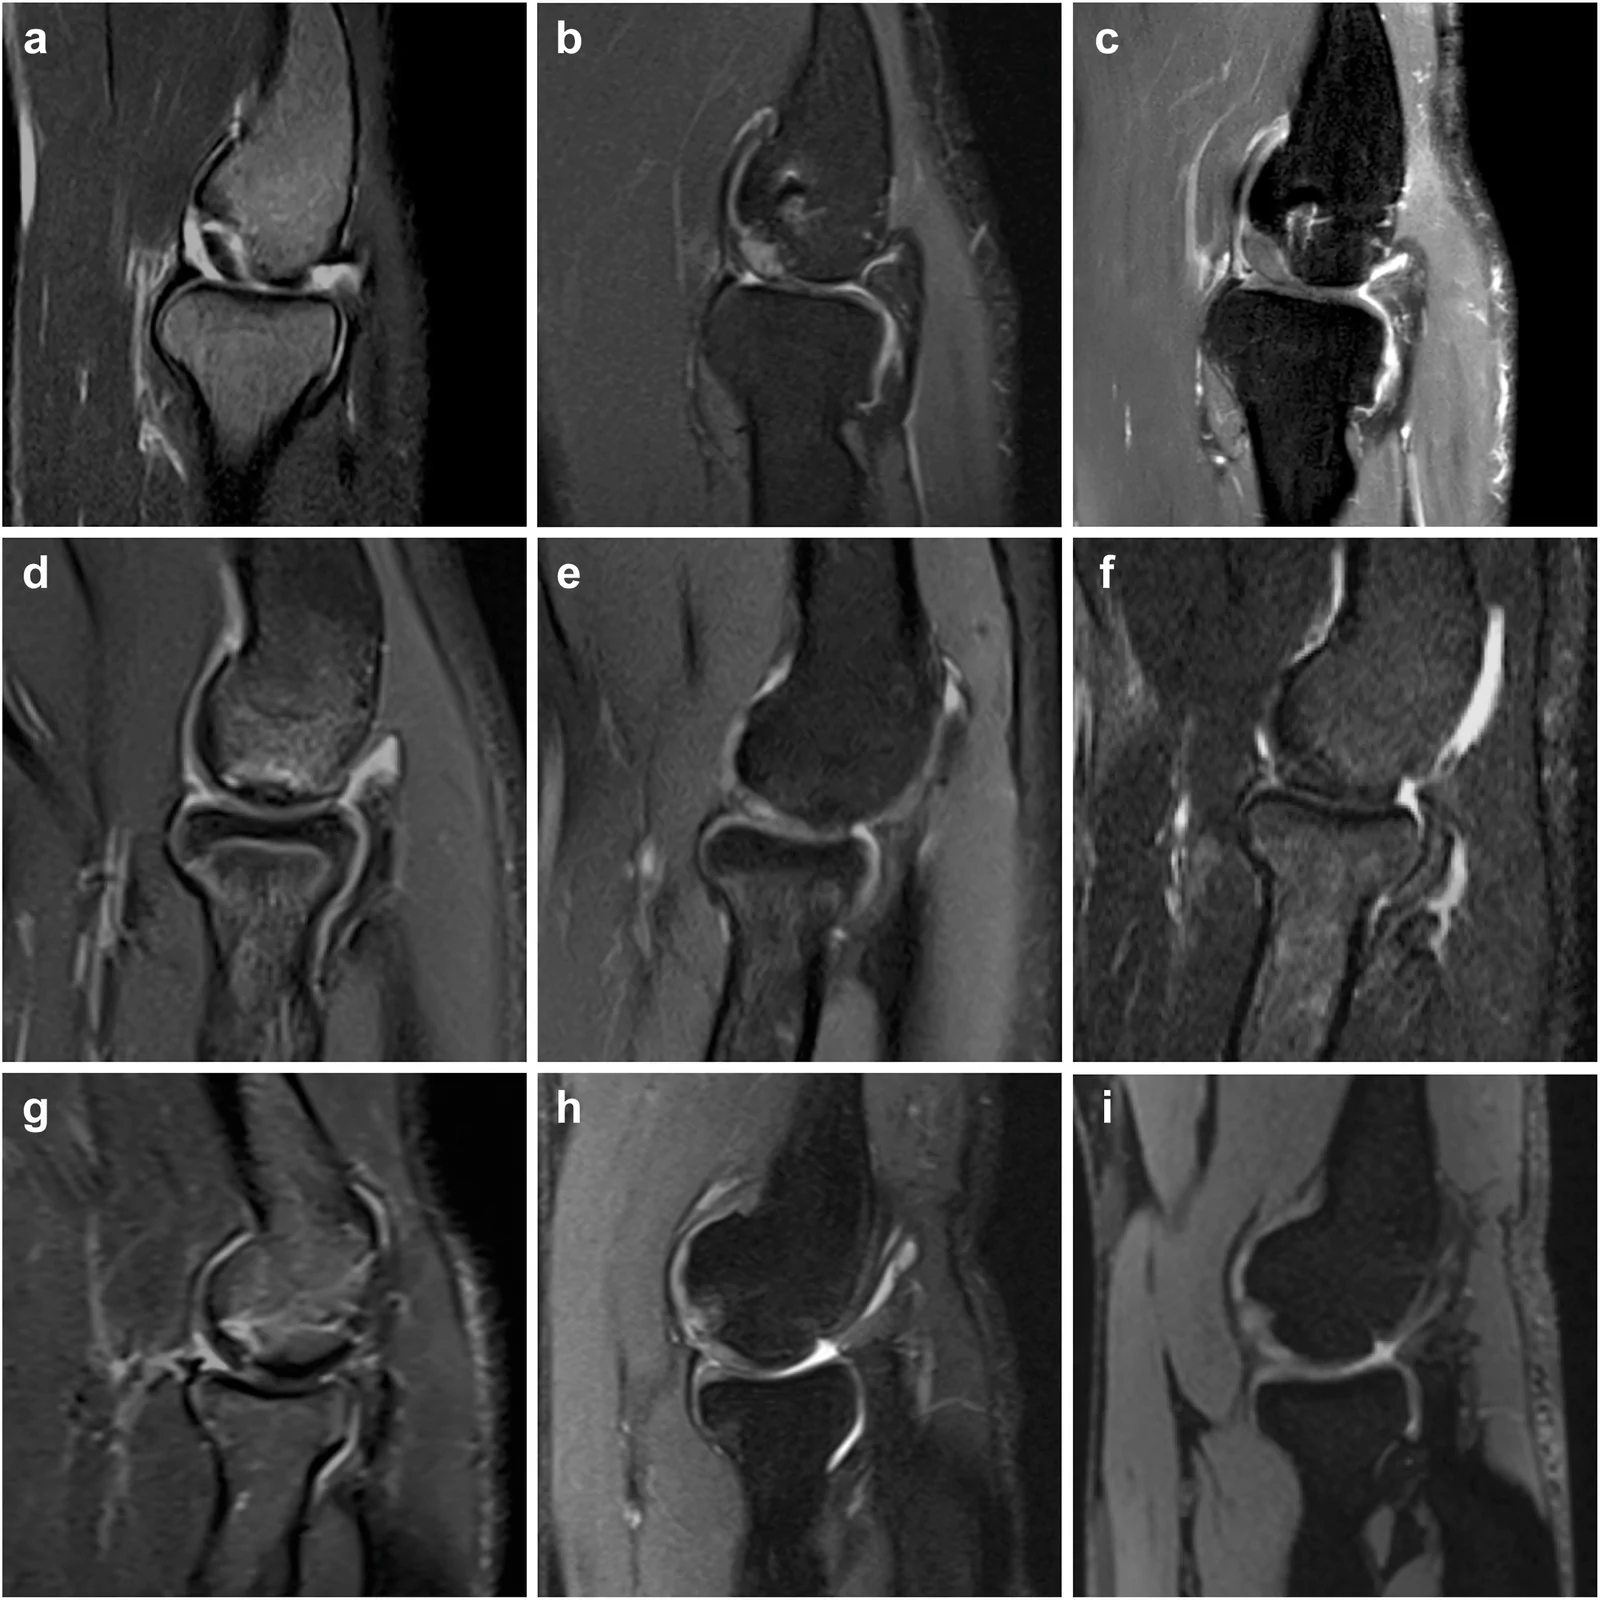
Sagittal MRI sections of 3 representative patients, each displayed in a single row, demonstrating the cartilage defect of the humeral capitellum pre-operatively (a, d, g) and the repair tissue at T2 (b, e, h) and T3 (c, f, i). *MRI*, magnetic resonance imaging.
